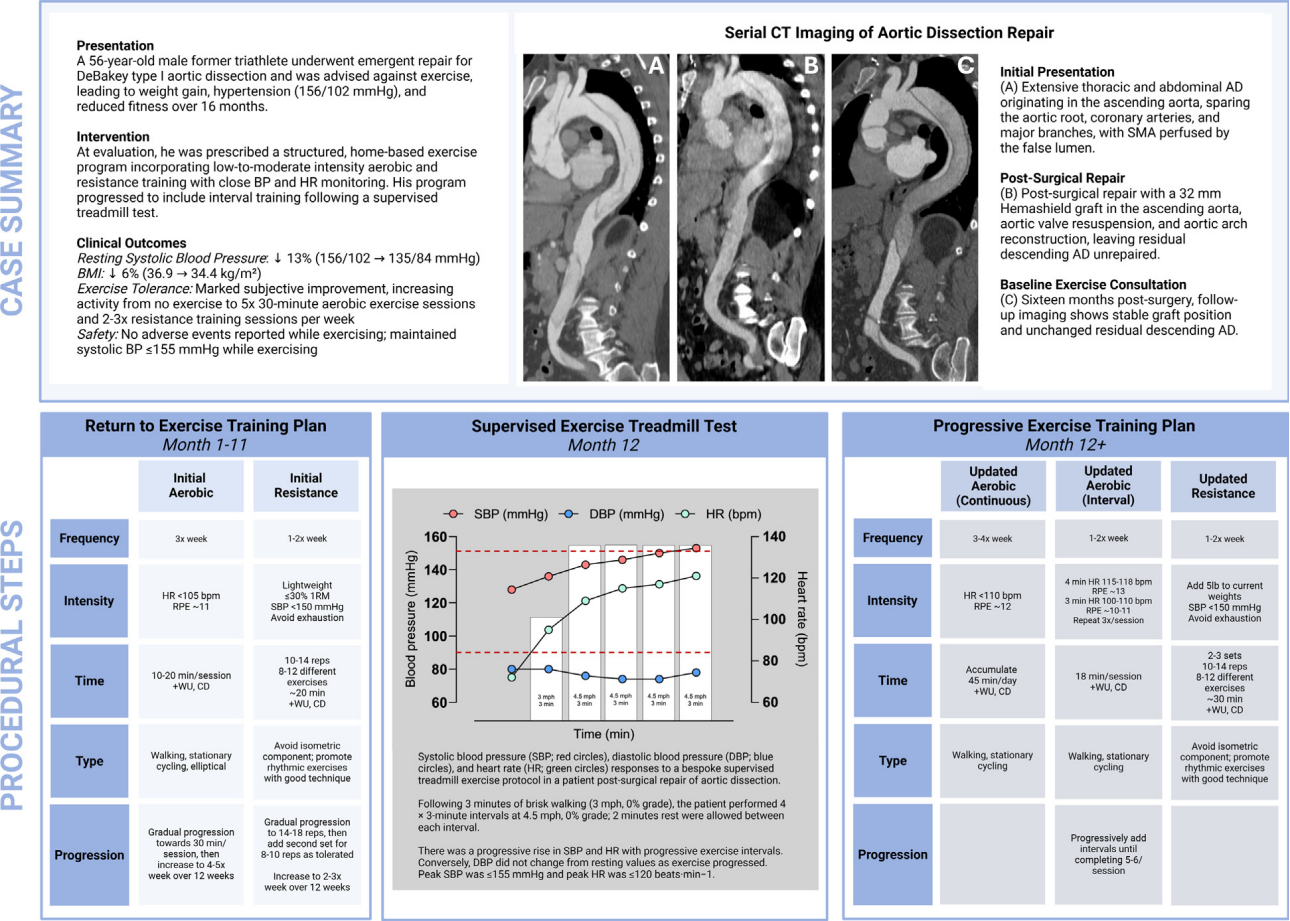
Case Summary, Serial Imaging, and Procedural Steps of Return to Exercise 1 RM = 1-repetition maximum; BMI = body mass index; BP = blood pressure; CD = cool-down; CT = computed tomography; DBP = diastolic blood pressure; HR = heart rate; RPE = rating of perceived exertion; SBP = systolic blood pressure; WU = warm-up.
